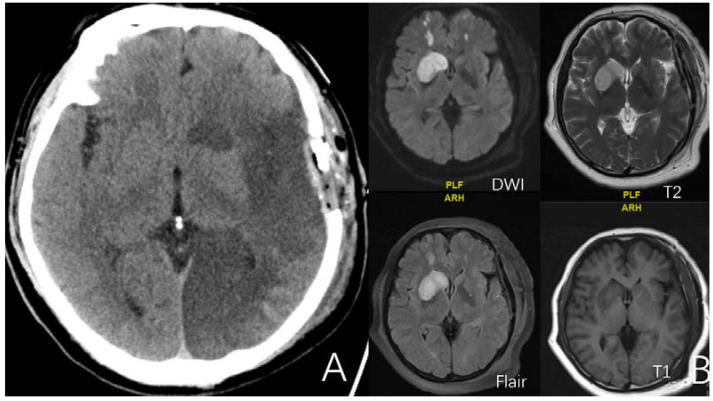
(A) is a 52-year-old female. CT showed massive infarct in the left cerebral hemisphere after surgery. (B) is a 52-year-old female. Four sequences of MRI exhibit the imaging features of minor cerebral infarction after surgery.
